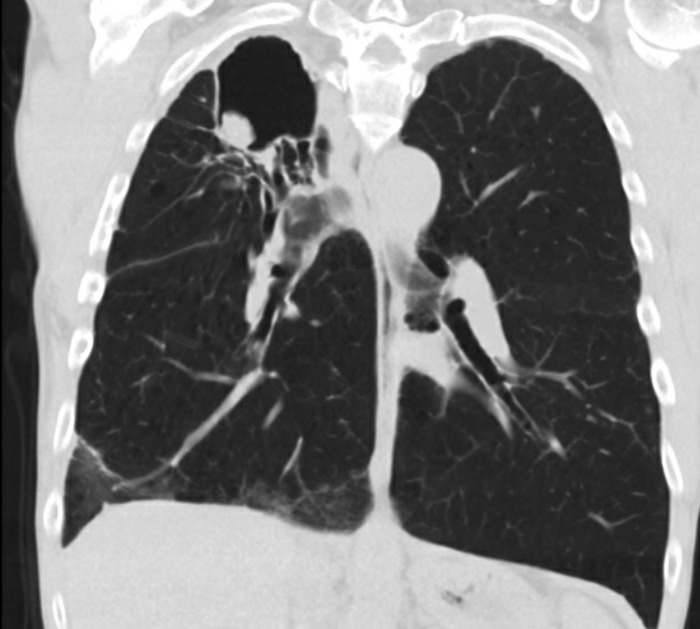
Follow-up chest computed tomography after 5 weeks, coronal view, shows stable borderline mediastinal lymphadenopathy.
